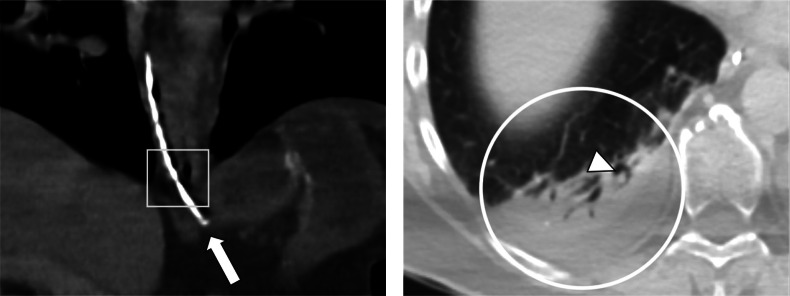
(Left picture) CT confirms the tip of the NGT inside the gastric cardia (arrow), only slightly distal to the lower oesophageal sphincter (square). (Right picture) Using lung windows, a right posterobasal consolidation and small adjacent pleural effusion (circle) were observed with intraluminal soft-tissue densities (arrowhead) in the supplying airways. This suggests aspiration as the cause.
